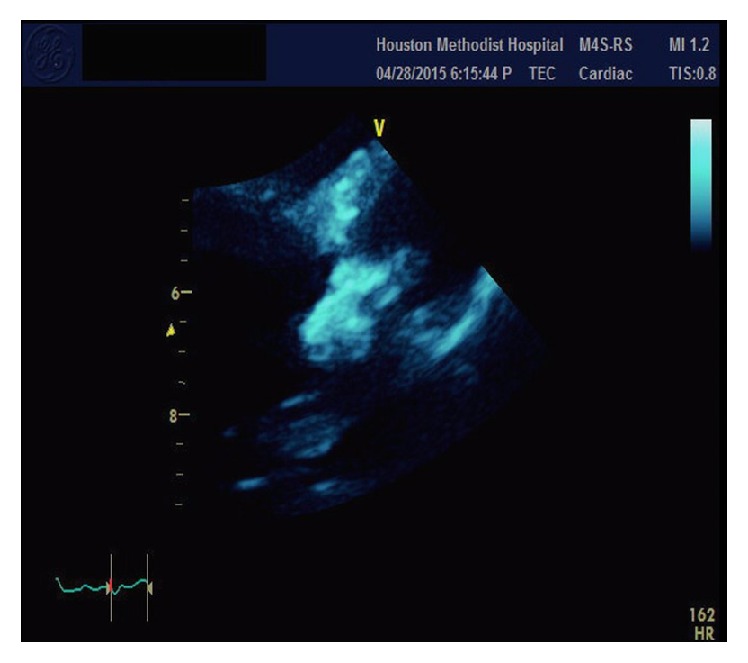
Aortic valve vegetation in parasternal long axis view.
